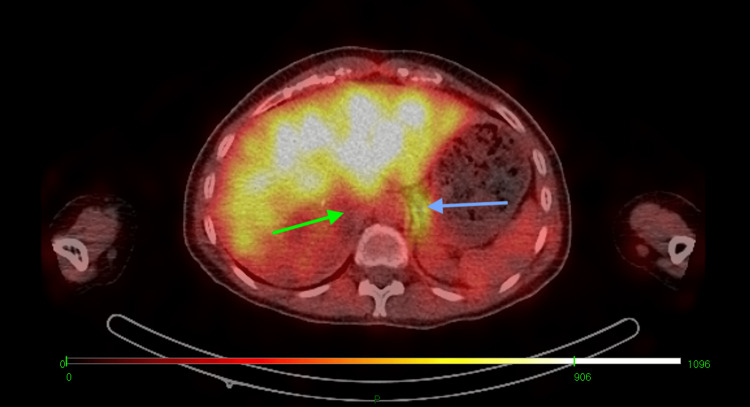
Postoperative metaiodobenzylguanidine (MIBG) scintigraphy showing absence of radiotracer uptake at the site of the resected right adrenal pheochromocytoma (green arrow), with normal physiological uptake in the left adrenal gland (blue arrow).
